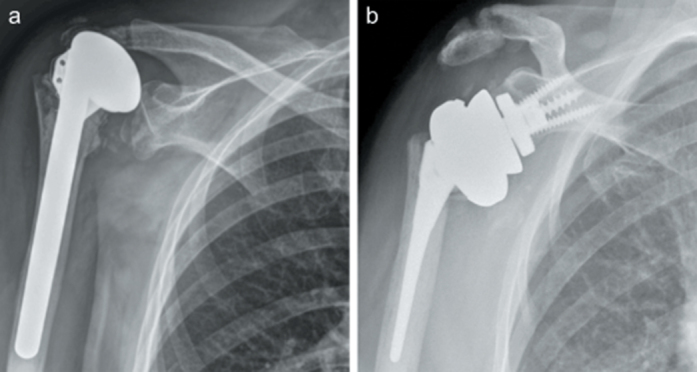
A postoperative 26th-month x-ray of a 65-year-old female patient treated with hemiarthroplasty for proximal humerus fracture reveals superior migration, acetabularization of the acromion, and glenoid erosion (a). RTSA using the cement-in-cement technique was performed after failed hemiarthroplasty (b).
